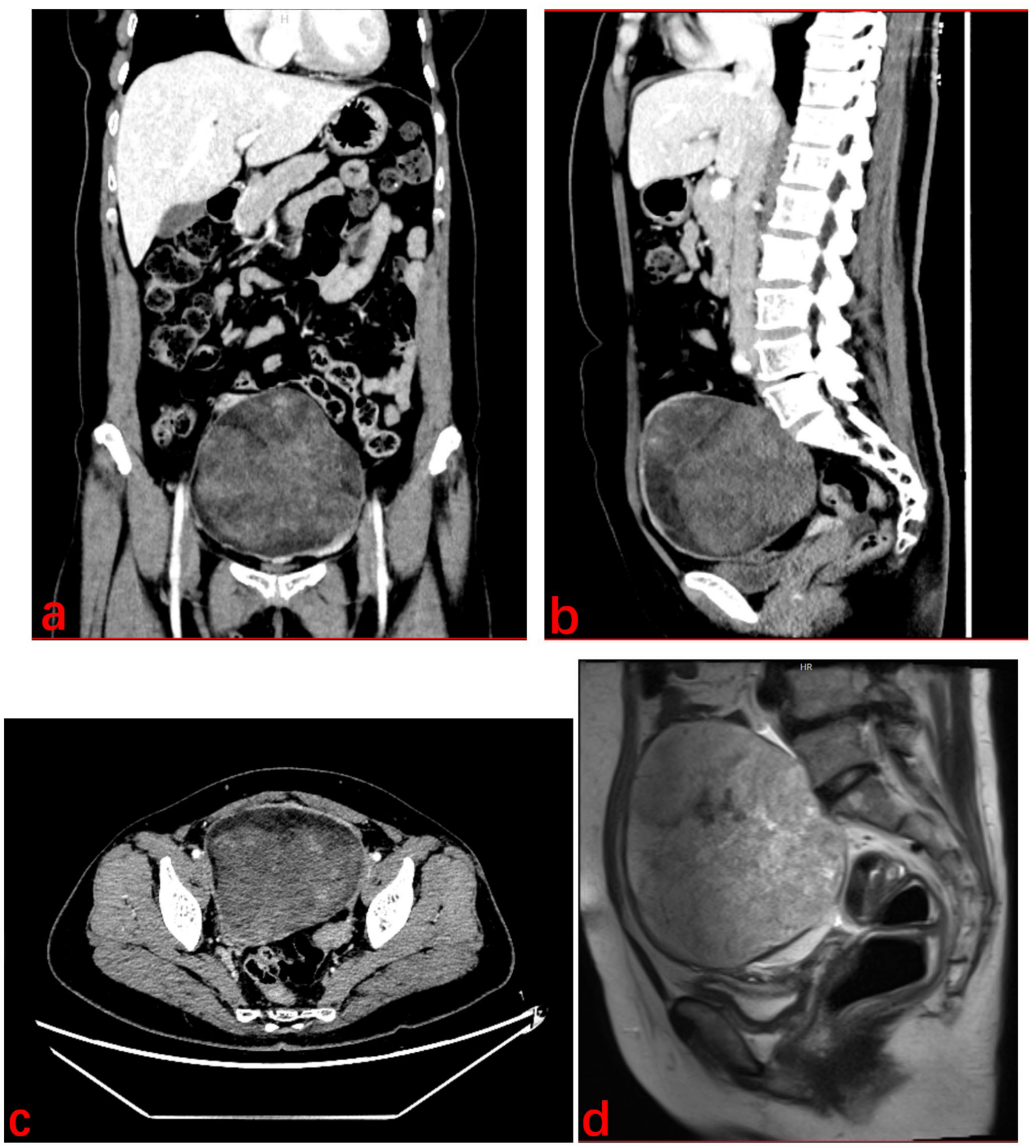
Abdominal CT. (A) Coronal section; (B) Sagittal section. (C) Cross section. (D) MRI of abdominopelvic mass.
